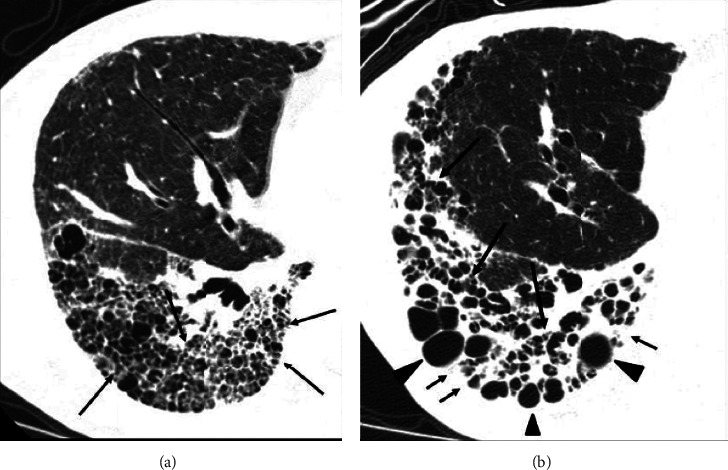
Sequential HRCT scans in a 74-year-old woman with fibrotic NSIP. (a) Initial CT scan shows traction bronchiectasis and bronchiolectasis (arrows) and cysts. The subpleural cysts seldom protuberate toward the extrapulmonary region. (b) Follow-up scan obtained 25 months later shows that many small cysts are collapsed and dense increased opacities have appeared. Traction bronchiectasis (long arrows) has progressed and some cysts have become larger (arrowheads). There are dense increased opacities in the subpleural region (short arrows).
